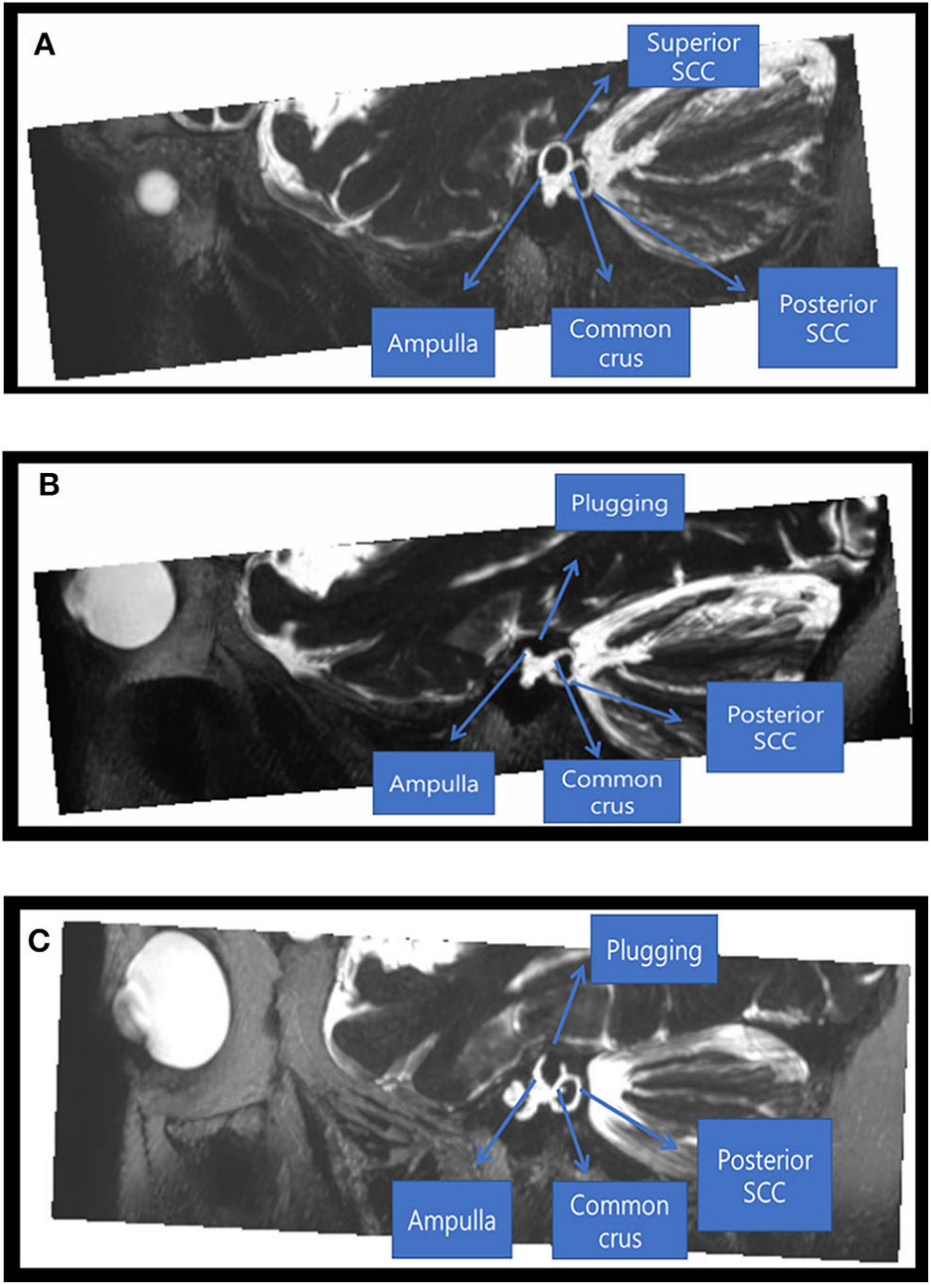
Radiological findings before and after canal plugging. Preoperative (A) and postoperative (B) images of a 46-year-old male patient who underwent canal plugging. (A) Maximum projection intensity reconstruction of oblique sagittal reformatted 3D T2-weighted volume isotropic turbo spin-echo acquisition (T2-VISTA) images (5 mm thick slab) shows T2-bright signal intensity in the patent superior semicircular canal lumen with dehiscence. (B) After plugging, the superior semicircular canal lumen was obliterated by filling the defect between the ampulla anteriorly and the common crus posteriorly. (C) Postoperative images of a 45-year-old female patient who underwent canal plugging. After plugging, the superior semicircular canal lumen was obliterated by filling the defect between the ampulla anteriorly and the common crus posteriorly.
